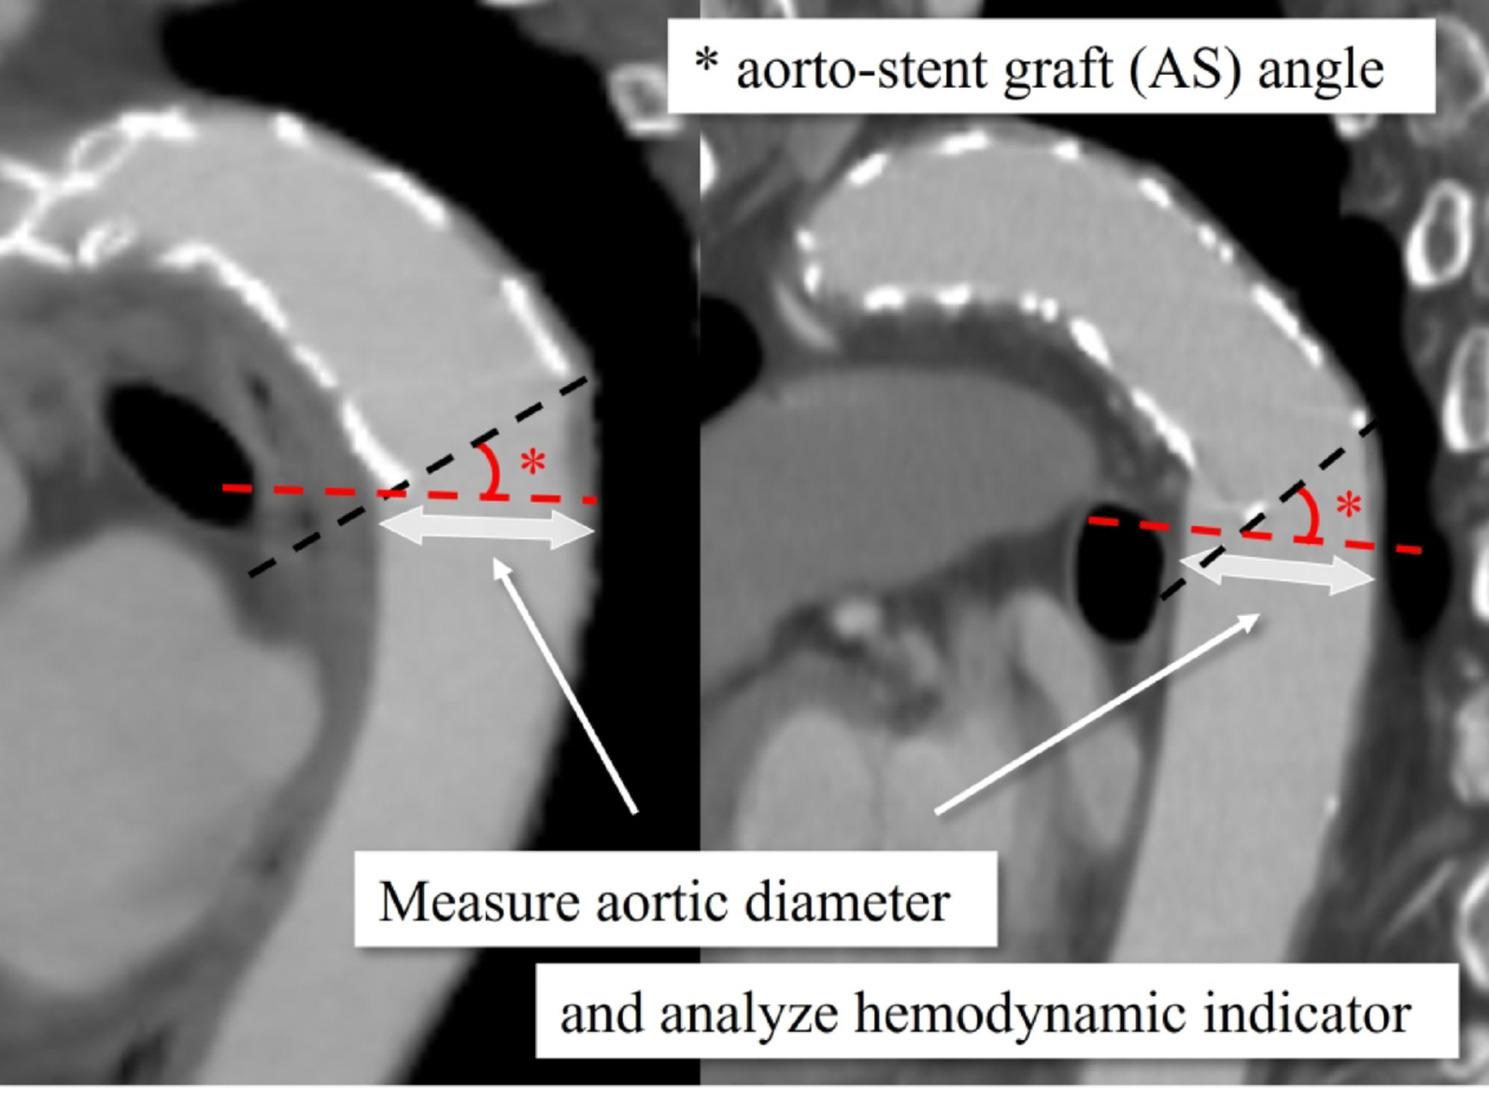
Definition of AS angle and measurement location. AS angle was defined as the angle between the aortic plane through the distal stent-graft end (red dashed line) and the distal stent-graft axis (black dashed line), shown by the asterisk. Aortic diameter change was measured as the difference in minor-axis diameter on the distal FET plane between the first follow-up confirming remodeling and the latest follow-up CT (white double arrow)
